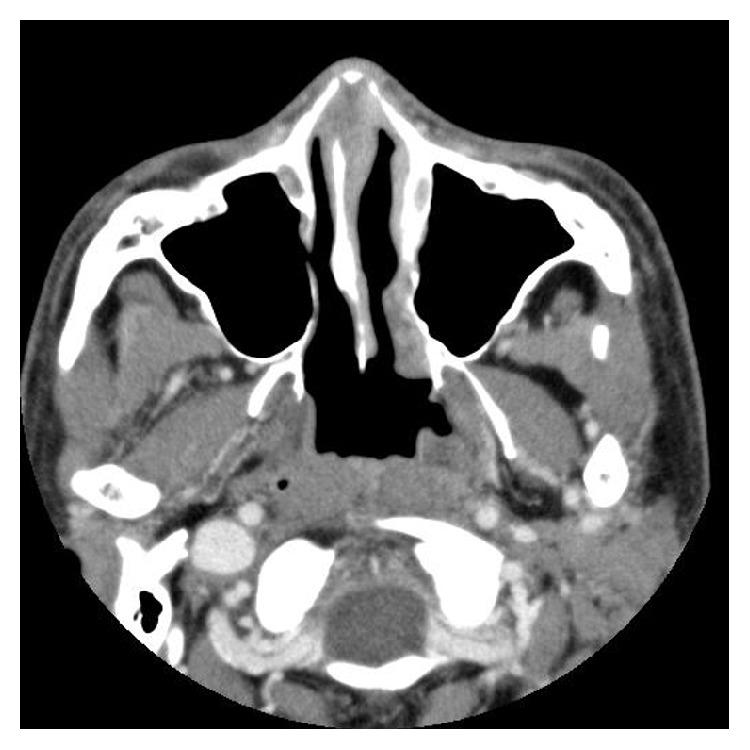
CT scan of a 36-year-old man with RP. The nasal septum is edematous and inflamed. CT, computed tomography; RP, relapsing polychondritis.
